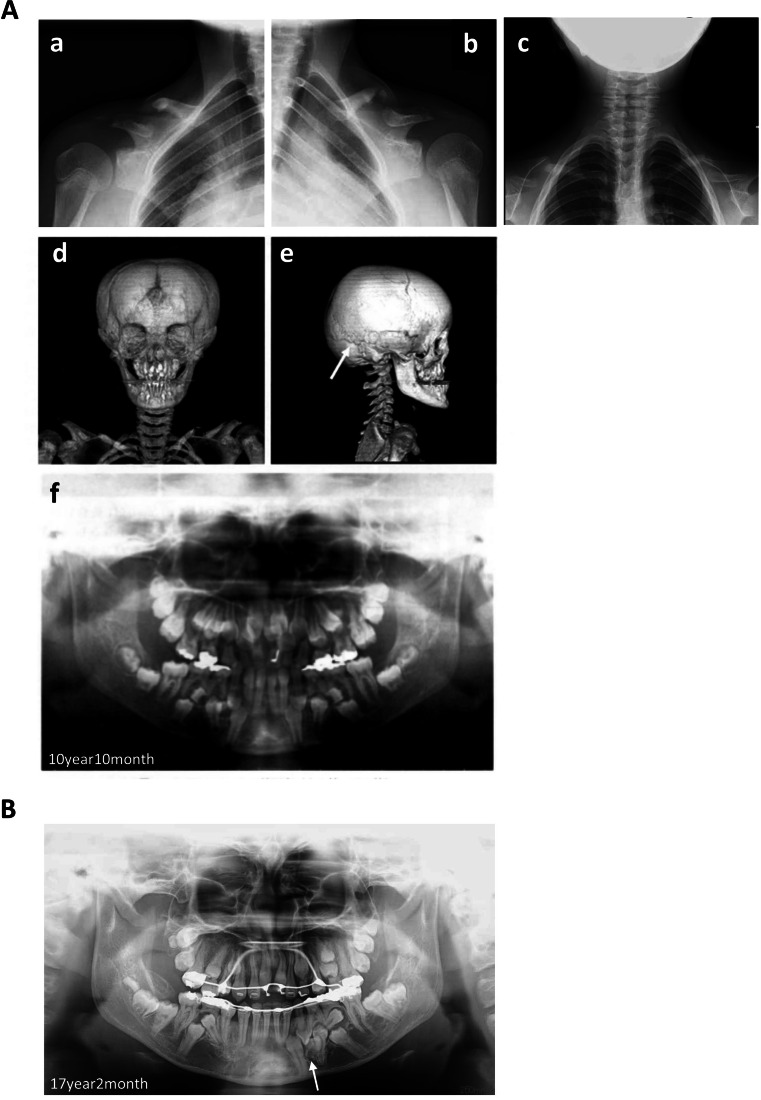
Typical morphologies from CCD donor (*A*) Typical radiological morphologies from CCD donor at the initial visit (*10 years10months*) (*a*) Chest radiograph showed partially absent clavicles. (*right side*) (*b*) Chest radiograph showed partially absent clavicles. (*left side*) (*c*) Chest radiograph revealed a cone-shaped chest, high position of the scapula, and bilateral clavicles were discontinuous. (*d*) Head computerized tomography showed open skull sutures, persistent open anterior fontanelle. (*e*) Head computerized tomography showed wormian bone and hypoplasia of the zygomatic arches. (*f*) Panoramic view showed delayed eruption of permanent teeth and supernumerary teeth at 10 yr and 10 mo (initial visit). (*B*) Panoramic view showed retention of deciduous teeth, delayed eruption of permanent teeth and many impacted supernumerary teeth at 17 yr and 2 mo. We obtained supernumerary tooth of mandible (*arrow head*).
